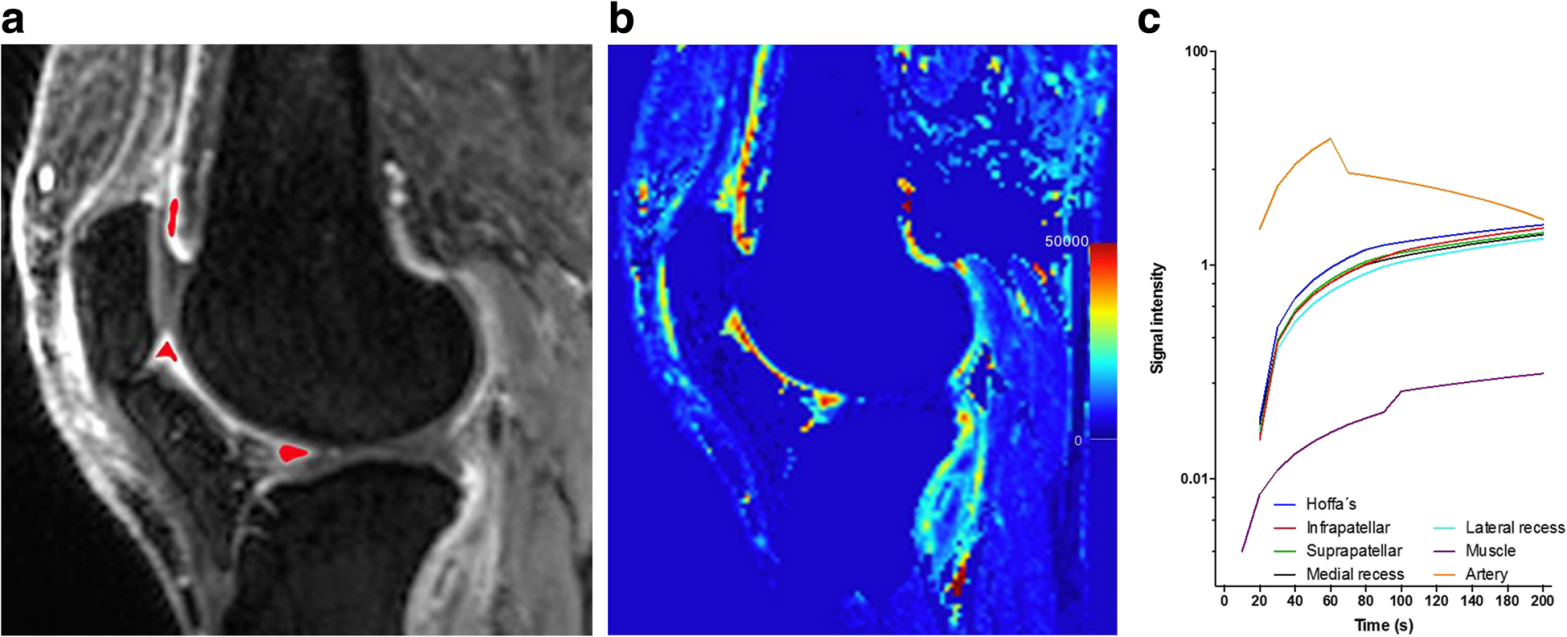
Dynamic contrast enhanced MRI. a Example of a sagittal T1-weighted dynamic contrast enhanced (DCE) image with demonstration of ROI placement in the last acquired image series at the most posterior border of Hoffa’s fat pad, adjacent to the inferior patellar pole and at the prefemoral fat pad. Regions of interest (ROIs) were centered within the synovial tissue, joint effusion or other articular structures were not included. b iAUC based calculation of parametric maps over time (color-coding represents arbitrary units (a.u.) of the iAUC from dynamic contrast-enhanced MRI ranging from blue to red (0–50.000 a.u.). c Typical enhancement curves from a single patient for the different ROIs show steep enhancement between 20 and 40 s followed by continued but much slower enhancement after 40 s
